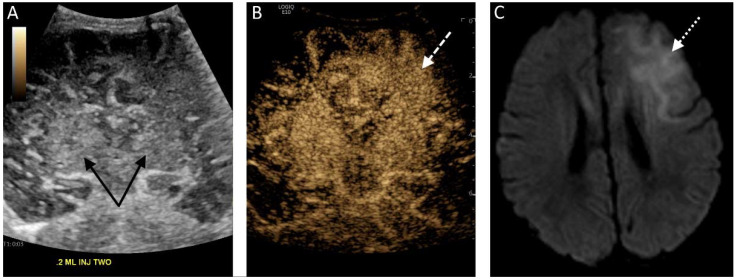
(A) Midcoronal grayscale ultrasound image of a 2 day old newborn’s brain through the deep gray nuclei (black solid arrows). (B) The contrast-enhanced image acquired 5 s after a second bolus injection in the same plane demonstrates enhancement of the bilateral deep gray nuclei but also asymmetric hyperenhancement of the left frontal lobe (white dashed arrow), representing luxury perfusion in an area of infarct. (C) A diffusion-weighted MRI sequence confirms the presence of diffusion restriction in the left frontal lobe (white dotted arrow) consistent with left frontal infarct.
